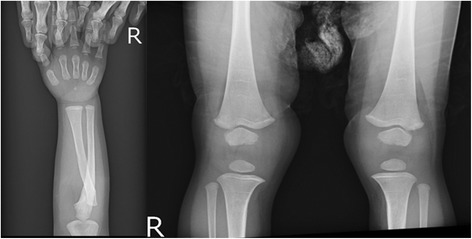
Radiographic image of the wrist and knee in the patient. No rachitic changes were observed in metaphysis
